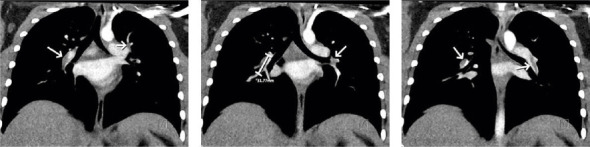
Images from CT-chest on POD3 demonstrating partially occlusive filling defects consistent with pulmonary embolism.
